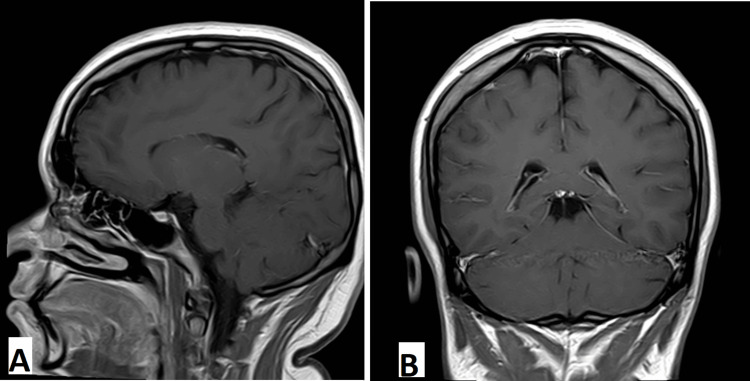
Normal T2-weighted MRI of the brain with contrast in sagittal view (A) and coronal view (B)
